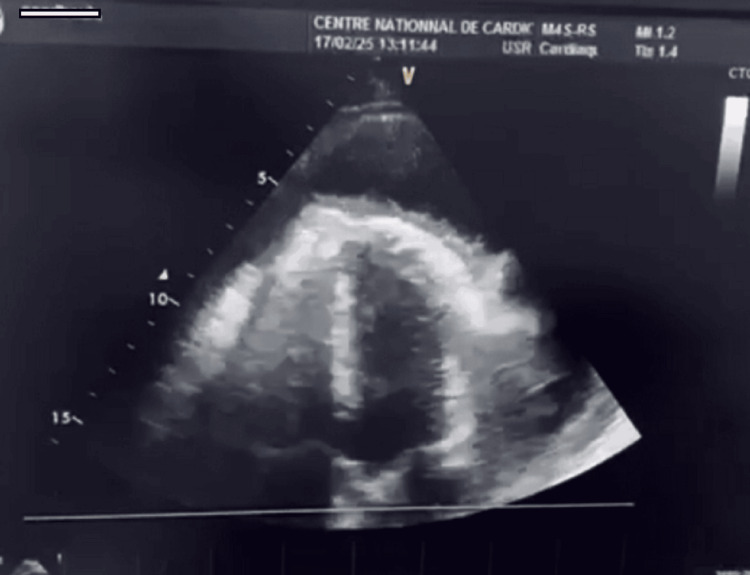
Transthoracic echocardiography The image shows a large circumferential pericardial effusion measuring 24 mm with signs of impending compression of the right heart chambers
